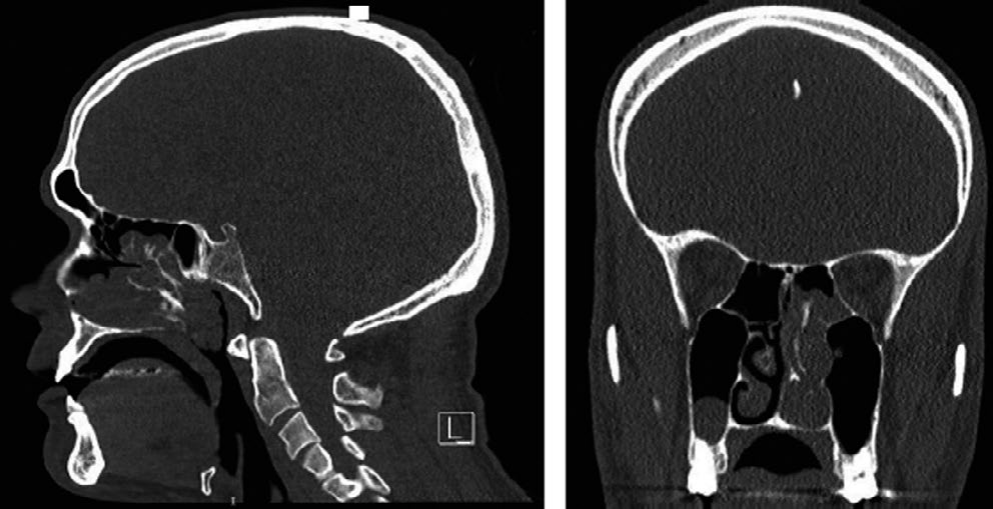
Noncontrast coronal and sagittal CT scans showing central clustered densities inside the posterior ethmoidal cells and the left nasal cavity. These structures are embedded in a polypoid tissue that extends from de left nasal cavity to nasopharynx and left posterior ethmoidal cells
